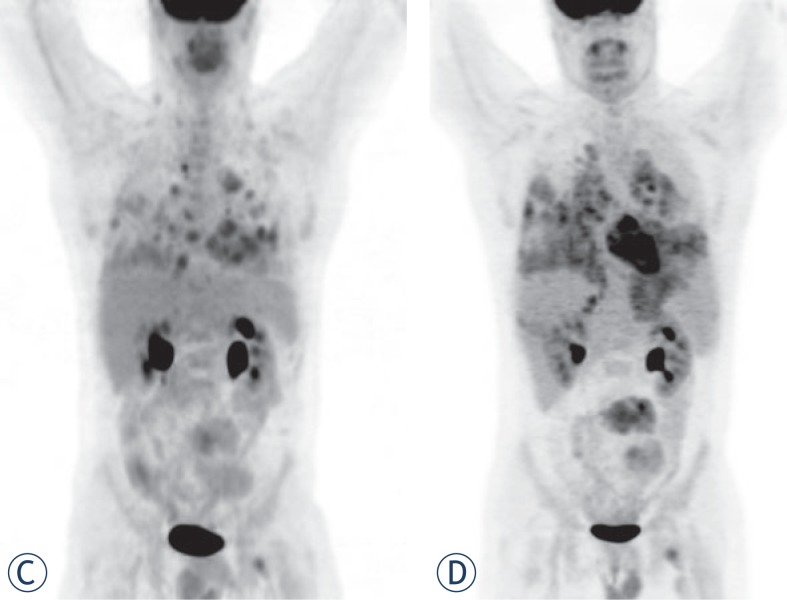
FDG PET study on October 2008 demonstrating tumor burden. (D). FDG PET study on December 2008 shows rapid evolution of tumor burden from October 2008.
